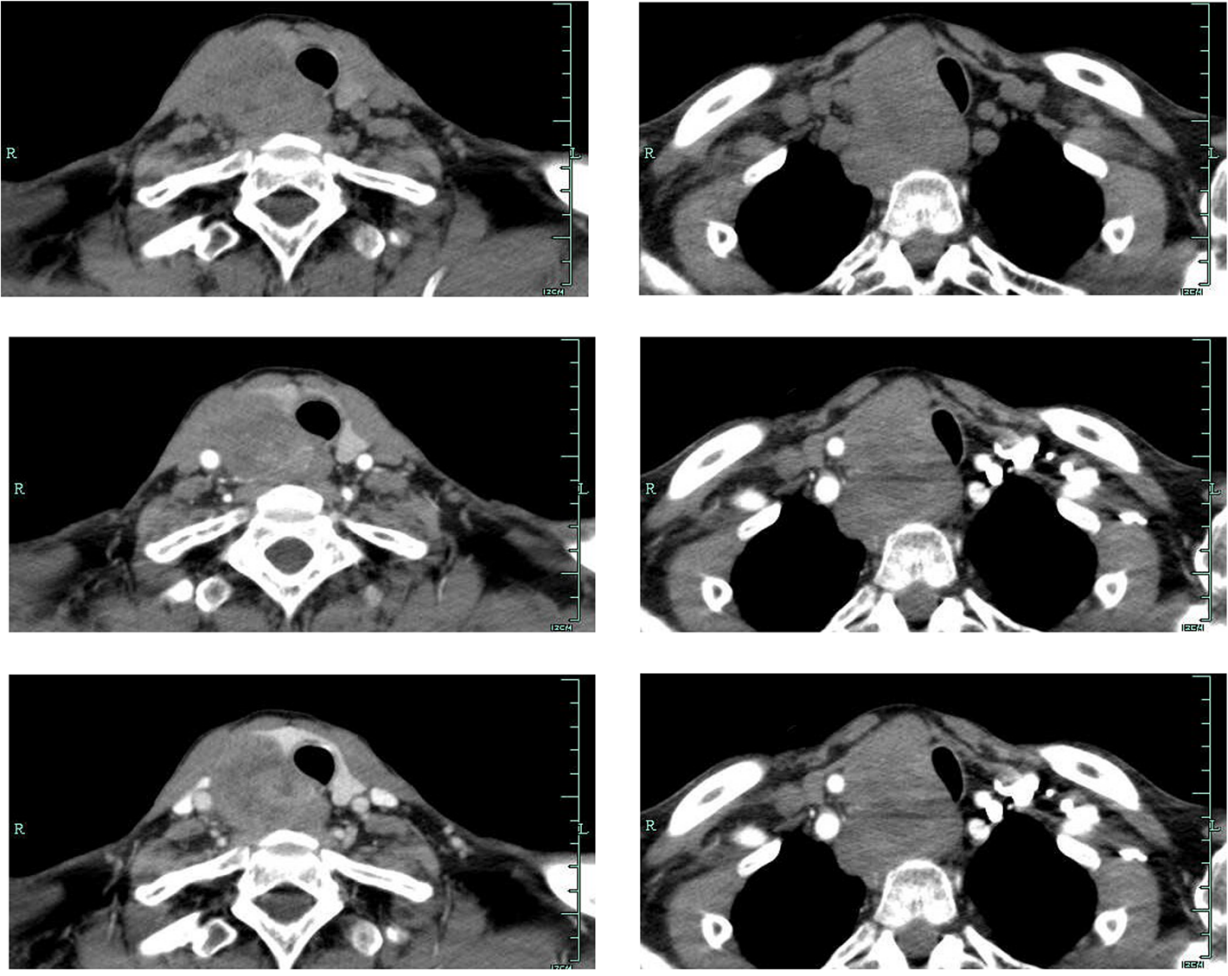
CT showed a large space-occupying lesion in the right thyroid region with invasion of the esophagus and mediastinum
